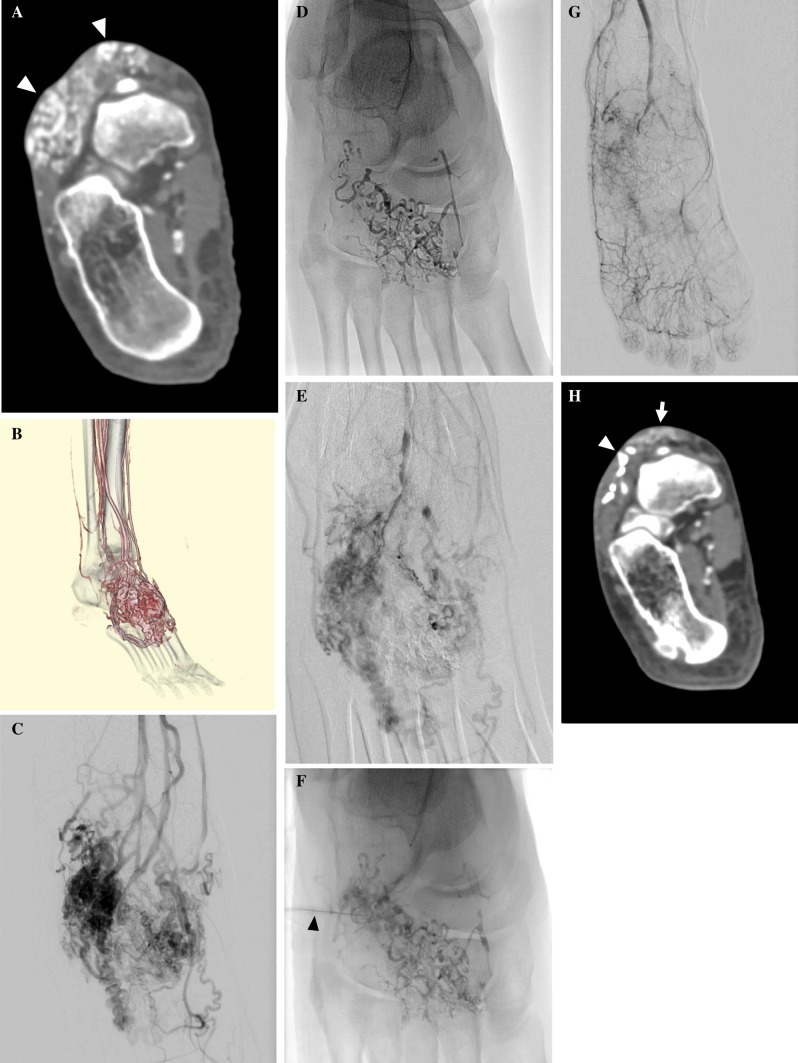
A 38-year-old woman with type IIIb AVM in the dorsum of the right foot with pulsating pain and swelling (Patient No. 17). A Contrast-enhanced CT shows a subcutaneous AVM with dilated abnormal vessels (arrowheads). B 3D-CT angiography demonstrates an AVM with dilated feeding arteries and draining veins. C Arteriography (frontal projection) demonstrates multiple feeders arising from the dorsal and plantar arteries and dilated draining veins. D Radiography after transarterial embolization from a feeding artery shows NBCA/lipiodol casts. E Arteriography after NBCA embolization demonstrates reduced flow of AV shunts. F Remaining AV shunts were punctured using a 27-gauge butterfly needle (arrowhead), and polidocanol sclerotherapy was performed under proximal compression using a tourniquet. G Arteriography after embolization and sclerotherapy shows almost complete exclusion of AVM. H Contrast-enhanced CT 3 years after treatment shows some remaining NBCA/Lipiodol casts (arrowhead) in the AVM and improvement in soft tissue swelling with shrinkage of subcutaneous veins (arrow). The patient has had no recurrence of pain in the long-term after the treatment. The therapeutic outcome was PR
